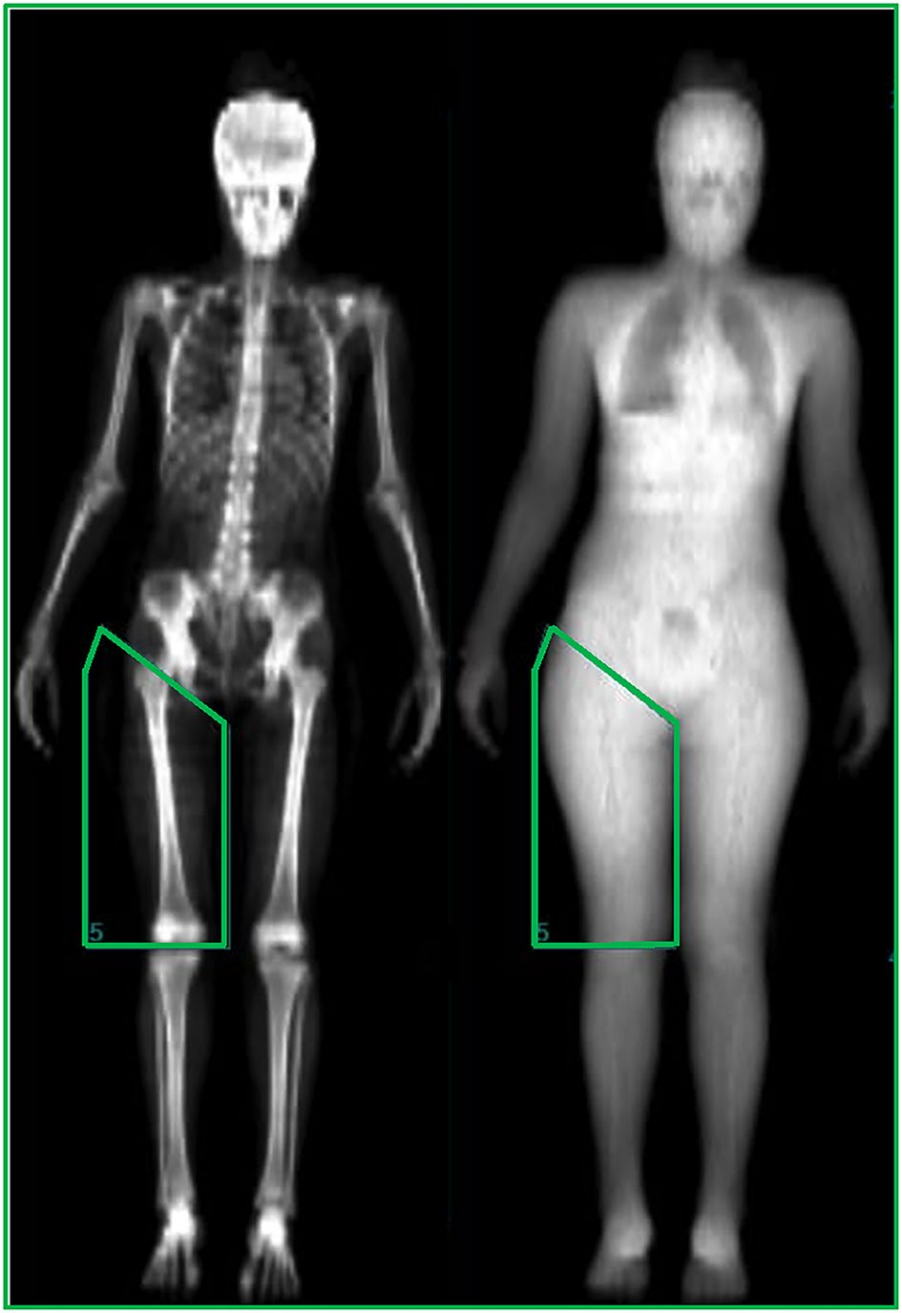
Dual-energy X-ray absorptiometry (DXA) image showing a full body X-ray scan from a single representative participant. Green traces show the thigh-specific measures that were performed using straight line borders (from the head of the femur to the tibial edge) to form a “thigh box” as a region of interest
